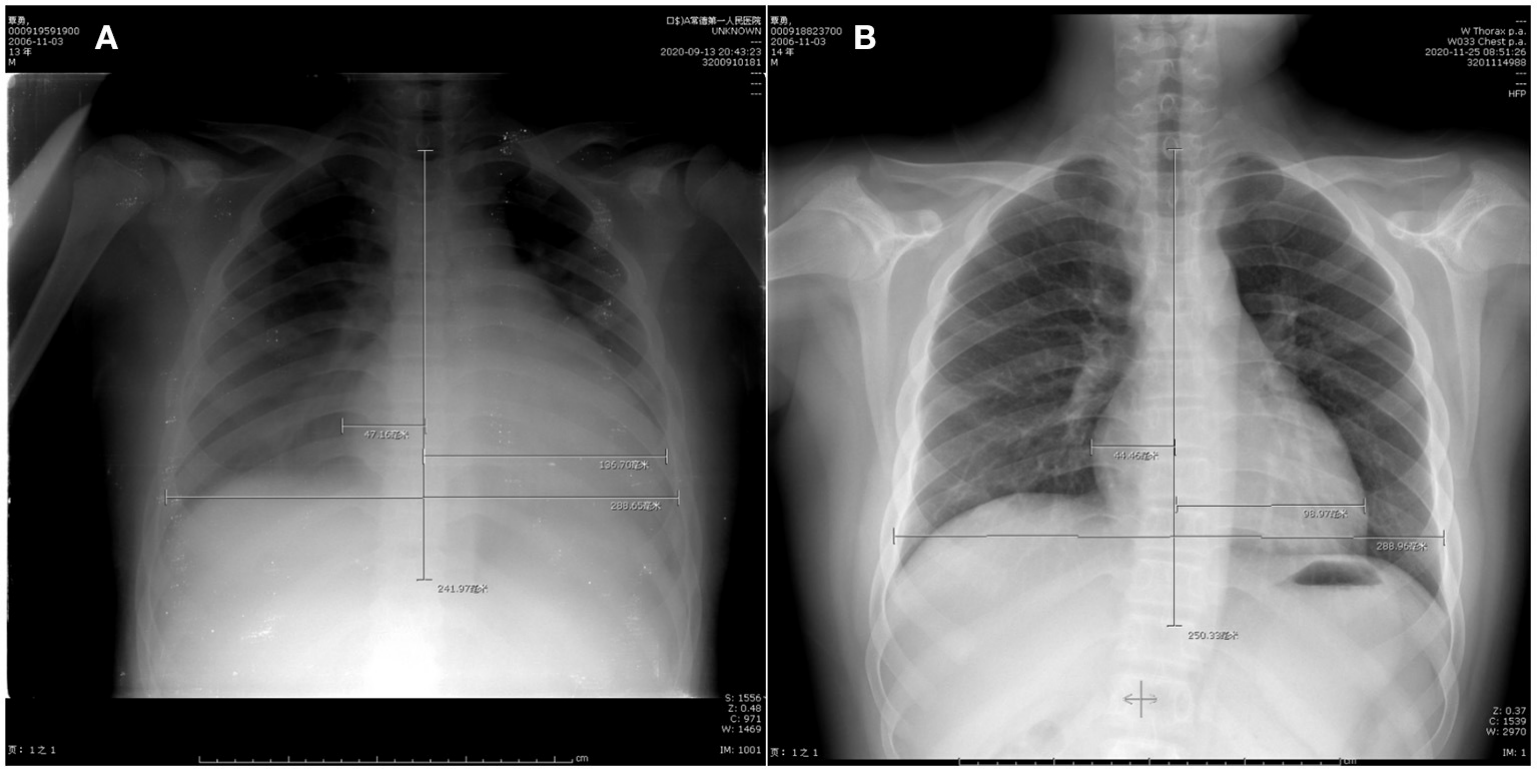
Chest radiographa (A) before operation, cardio-thoracic ratio 0.64; (B) 2 months after operation, cardio-thoracic ratio 0.50.
